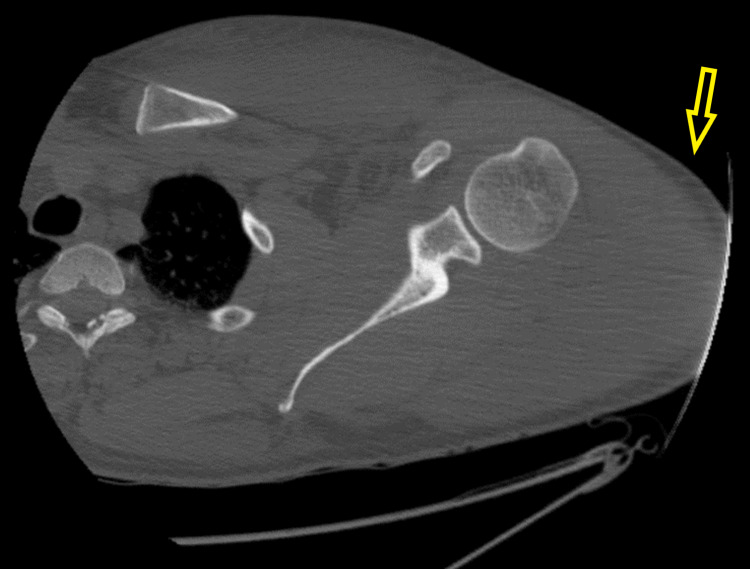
CT scan of the left upper extremity showing diffuse edema (yellow arrow) within the left shoulder compartment.
